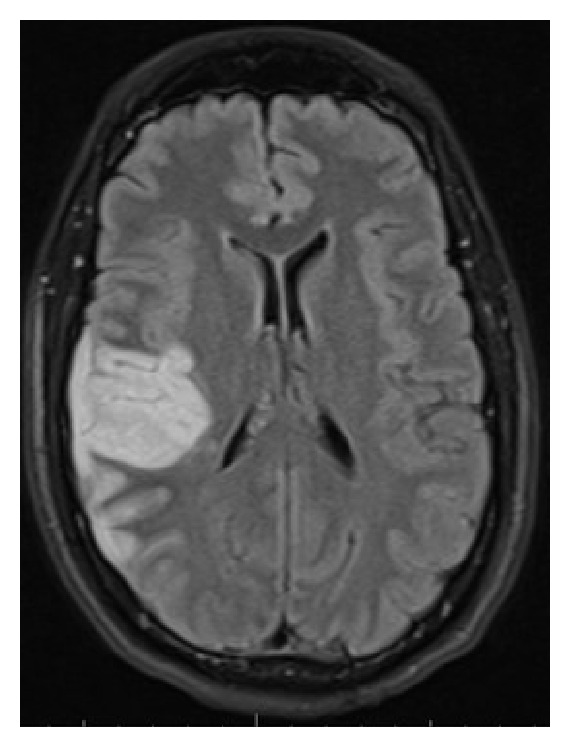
MRI of the brain with acute ischemic infarct in the right lobe.
